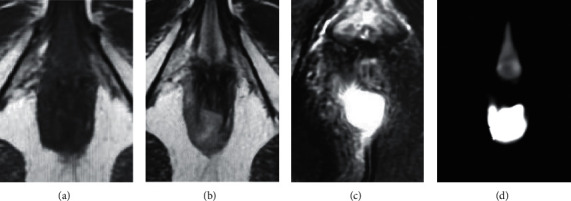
Different MRI-sequence images of the patient with perianal abscess. A is the image of T1WI, B is T2WI, C is DWI, and D is ADC (male, 44 years old).
